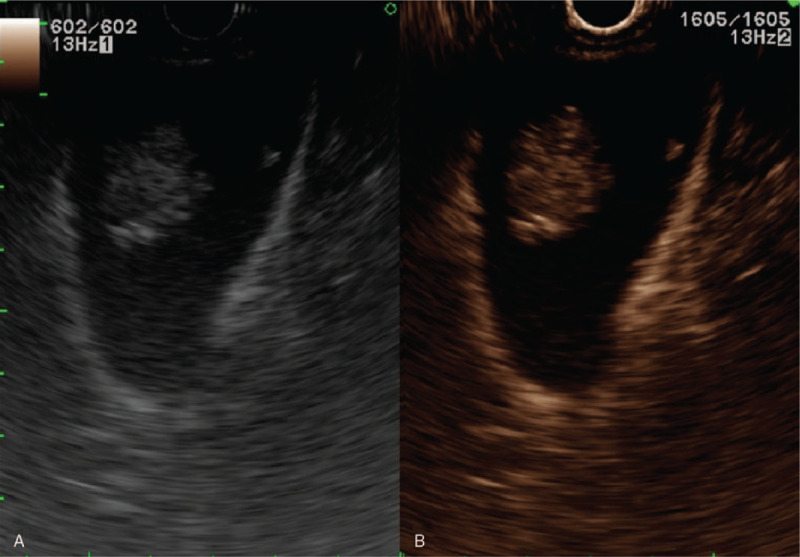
The gallbladder mass measures 24 mm and shows enhancement on contrast-enhanced ultrasonography (CEUS). (A) Gray-scale image. (B) CEUS image of the lesion.
